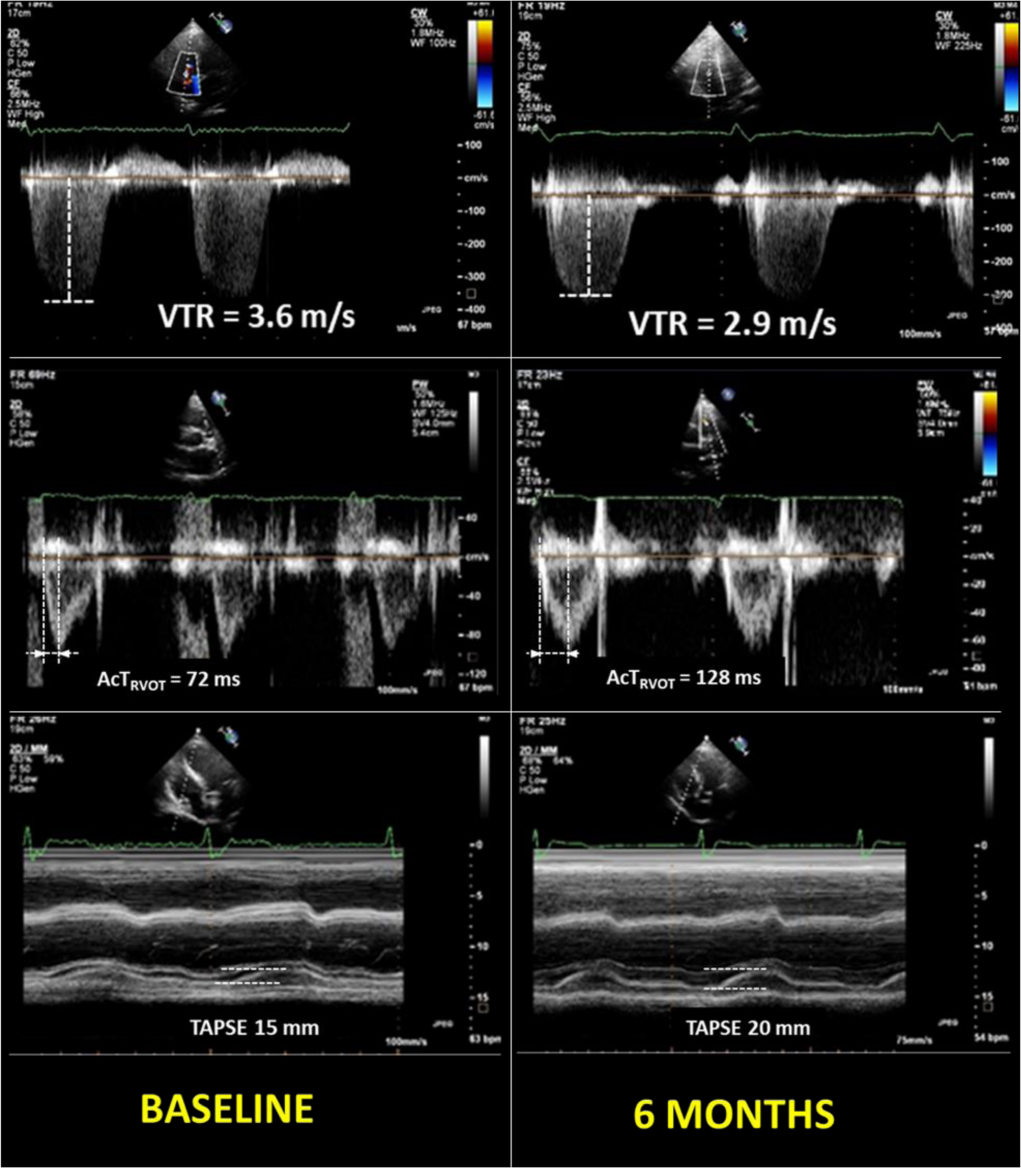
An example of Doppler peak tricuspid regurgitation and right ventricular outflow tracings, and tricuspid annular plane systolic excursion in a study patient with HFpEF at baseline (left panel) vs. after 6-month sildenafil therapy (right panel). Sildenafil therapy was associated with improvements in pulmonary artery systolic pressure (a decrease in tricuspid regurgitation velocity, TVR), pulmonary vascular resistance (an increase in the time to peak velocity of right ventricular outflow velocity, AcT_RVOT_), and right ventricular systolic function (an increase in tricuspid annular plane systolic excursion, TAPSE)
